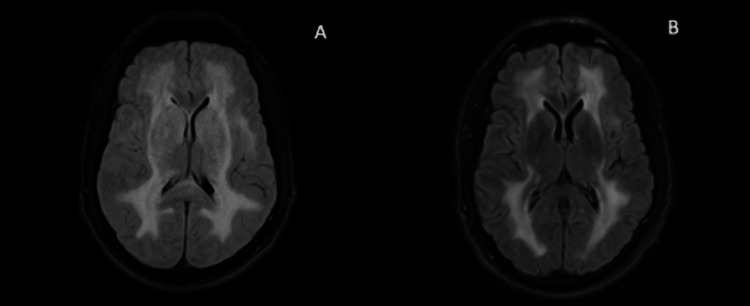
Comparison between MRI images (A) Initial MRI FLAIR with diffuse leukoencephalopathy. (B) Repeat MRI FLAIR nine months following initial presentation that demonstrates interval resolution of white matter signal change, especially in the basal ganglia and thalami. FLAIR: fluid-attenuated inversion recovery
